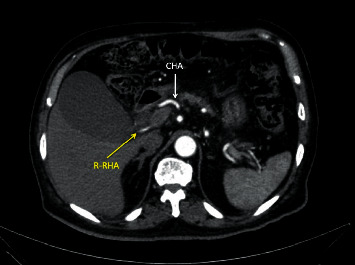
Axial CT images of a patient with a type 3 variant. The normal common hepatic artery (CHA) can be seen coursing laterally in a normal route (white arrow). The replaced right hepatic artery (R-RHA) can be seen coursing toward the right liver posteriorly in the hepatoduodenal ligament, behind the portal vein.
